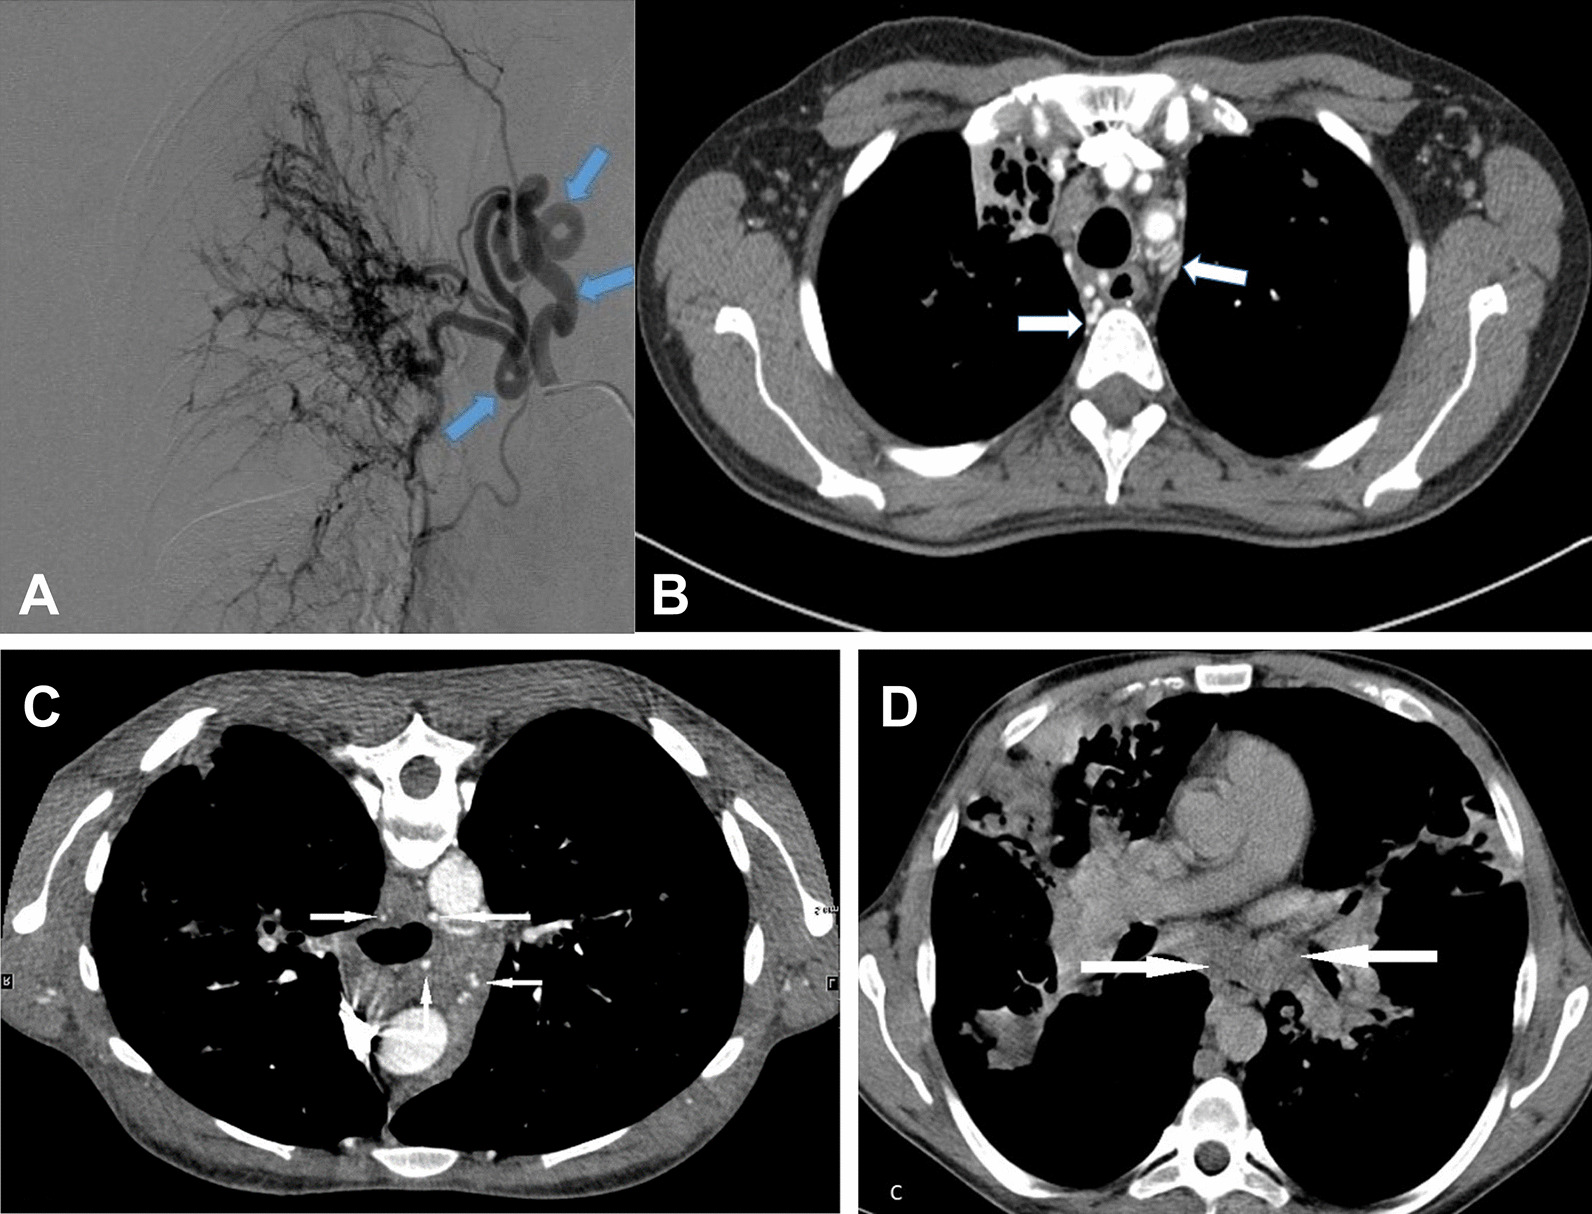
A B: Hypertrophy of bronchial arteries in the same patient can be seen in digital subtraction angiography (arrows in A) and in contrast enhanced CT scan (arrows in B). C: moderately hypertrophied bronchial arteries in a patient with end stage CF but no history of hemoptysis (arrows show bronchial artery). D: In this patient non-contrast-enhanced CT was performed and hypertrophied bronchial arteries cannot be distinguished from lymph nodes or other pulmonary vessels in the mediastinum (see arrows). Hypertrophy of bronchial arteries is easily missed in CT scans without contrast enhancement
